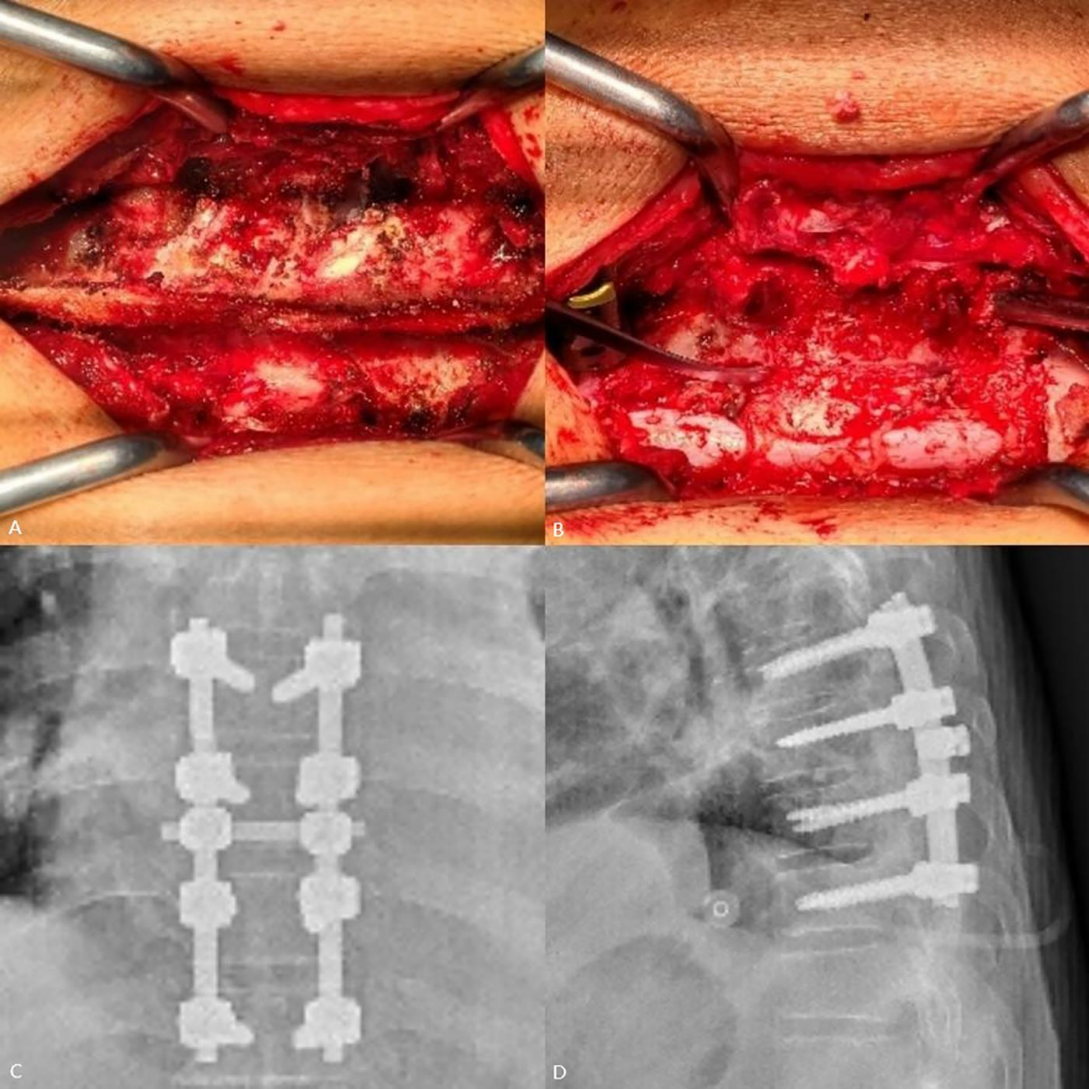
Intraoperative photos and postoperative imaging (A) intraoperative view: gypsum-like white particles (indicative of calcific or urate deposits) are present within the interlaminar space (indicated by the arrow). (B) Postdecompression: Gypsum-like white particle deposits in the left spinal canal cause spinal cord compression and deformation (indicated by the arrow). (C,D) Postoperative anteroposterior and lateral x-rays: demonstrating adequate decompression and restoration of spinal canal integrity.
